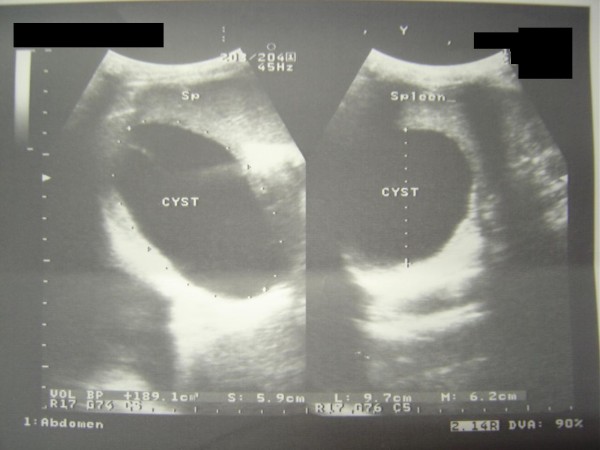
Case 1 – Hydatid cyst of the spleen. Ultrasound of the spleen showing a cystic lesion in the spleen.
